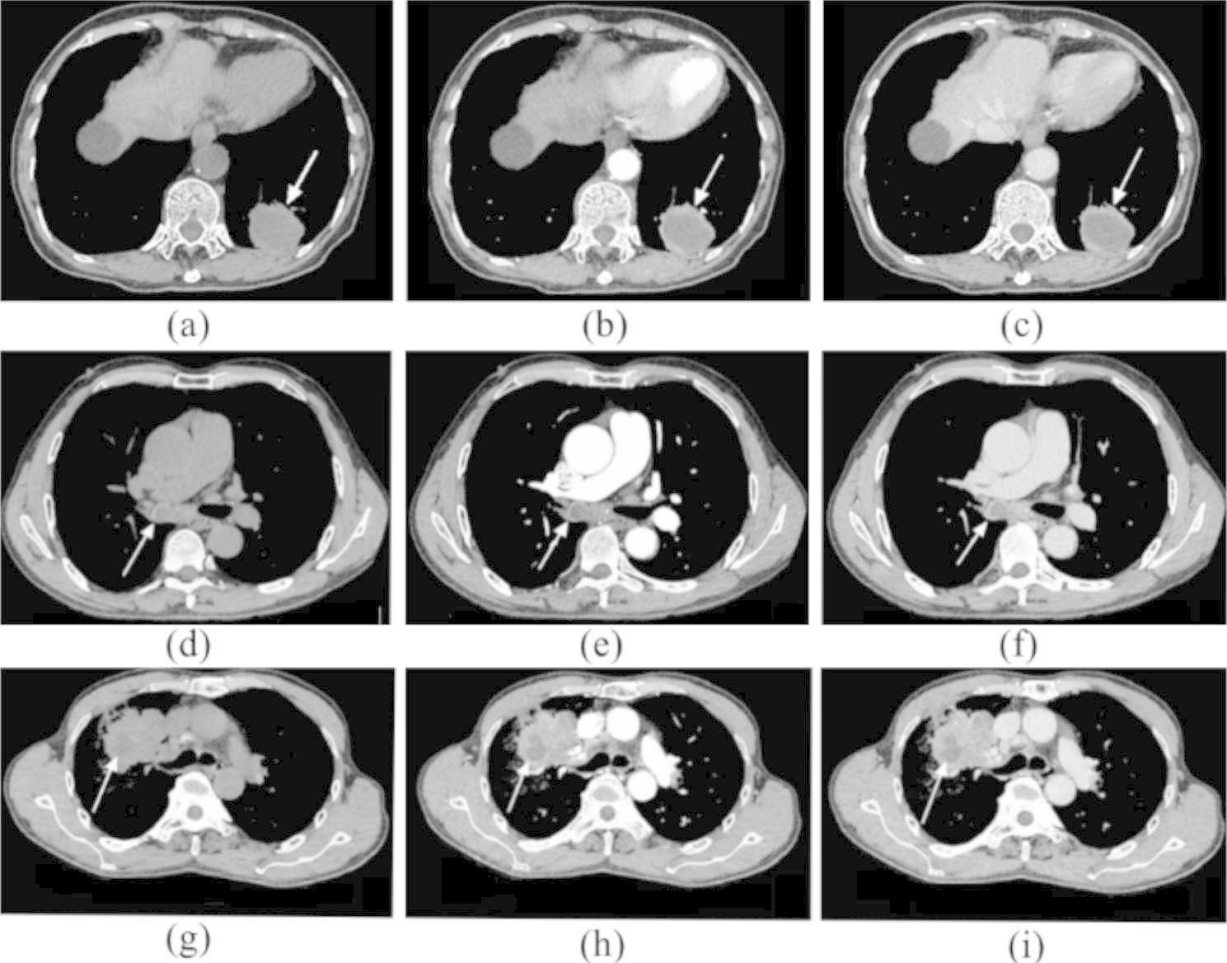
(a)-(c) A 68-year-old female with PSC in the left lower lobe (arrow). The LAA could be found at the central region of the lesion, with no enhancement on contrast-enhanced CT scans. In this case, the LAA ratio was 61%. (d)-(f) A 63-year-old male with PSC in the right lower lobe bronchus (arrow). Although the lesion is small, the LAA ratio is relatively large. The LAA ratio was 51%. (g)-(i) A 54-year-old male with adenocarcinoma in the right upper lobe. The LAA is seen within the tumor (arrow), but the LAA ratio is relatively small (19%)
